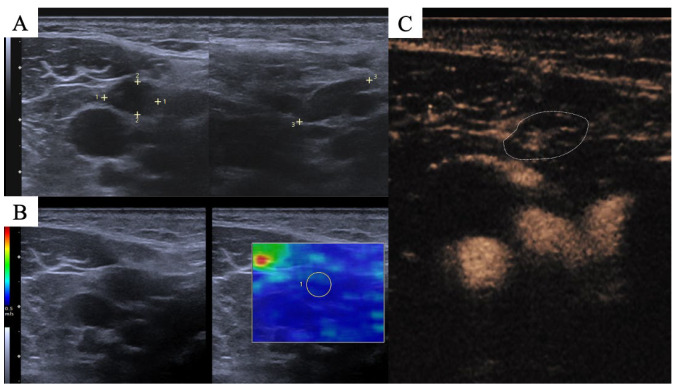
Representative example of ultrasound image evaluation. (A): B-mode US, CLNs were evaluated in two planes for their morphological appearance (size, shape, and borders; 1–3: diameter in each plane) (B): SWE, left side with B-mode US image and color bar (blue indicating soft and red indicating hard tissue), right side showing result of SWE assessment within the ROI (yellow circle marked with “1”). (C): Contrast inflow of a CLN at 15 s of a 90 s CEUS cine loop. The video was evaluated for perfusion pattern, distribution homogeneity and presence of necrosis.
